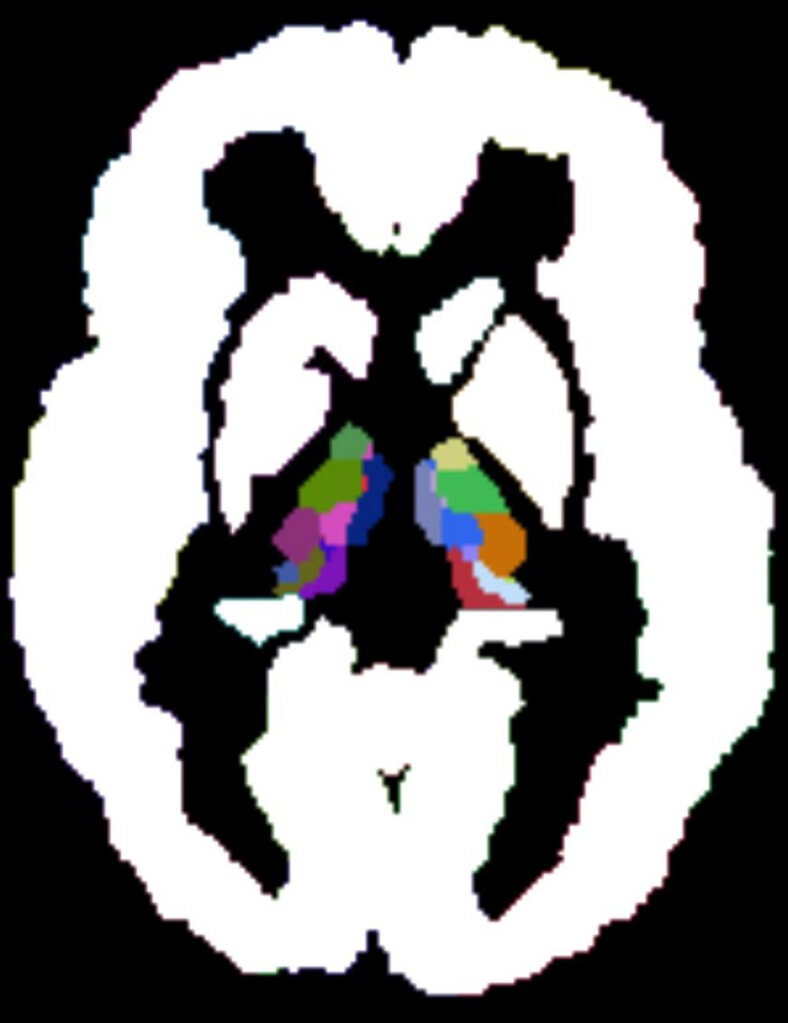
Segmentation of the thalamic nuclei. Automated segmentation of thalamic nuclei based on structural MRI data following aal3 atlas.
